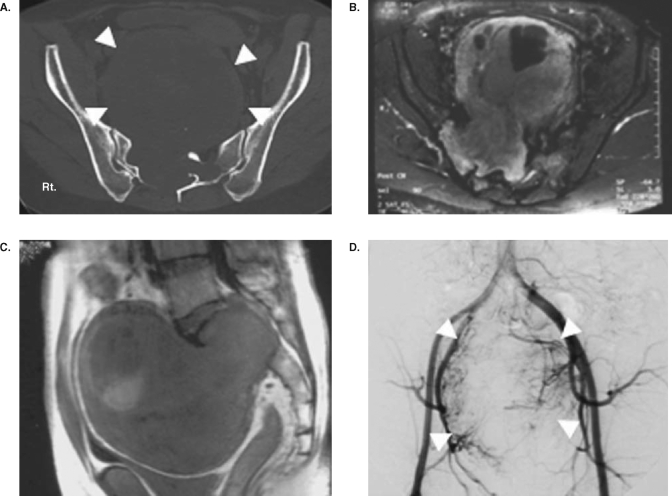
Case 2. CT (A) showing bony destruction of the sacrum and tumor outline (white arrow-head). Axial image (B) and sagittal image (C) of MRI showing the enormous tumor with heterogeneous signal intensity. Preoperative angiogram (D) showing a high vascularity of the tumor (white arrow-head) from both internal iliac arteries. The tumor was excised completely by a piecemeal excision with right S1 root sacrifice via the posterior and anterior approach.
